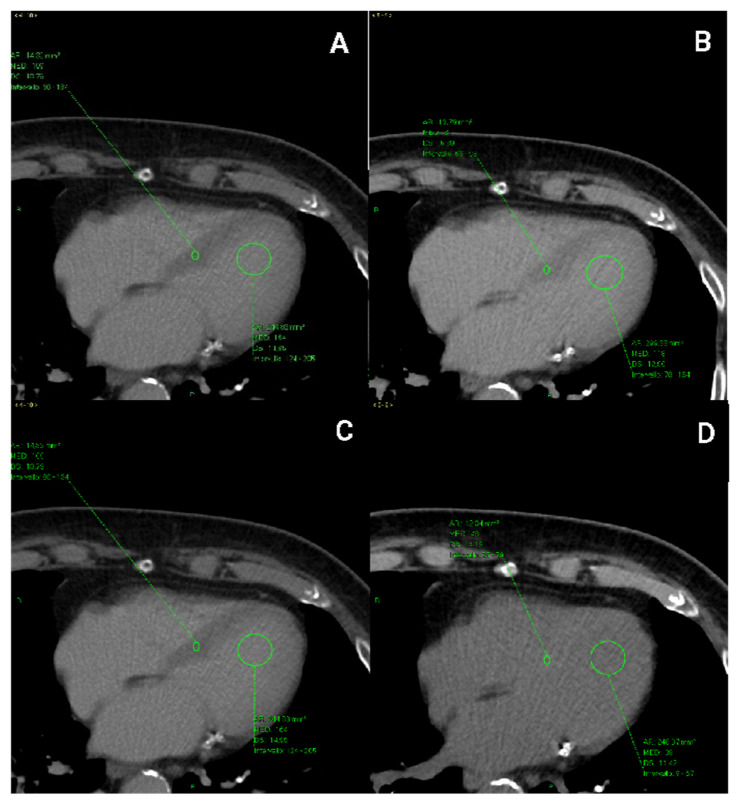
ROI (green arrows) located in the interventricular septum and left intraventricular blood pool in CT scans acquired (A) during the portal phase (1 min after contrast mean injection) and (B) in the delayed phase (5 min after contrast mean injection), respectively. ROIs used for ECV calculation in CT scans were acquired (C) in the pre-contrast phase, where the ventricular septum appears more hyperdense than the cardiac chambers (D) and in the portal phase.
